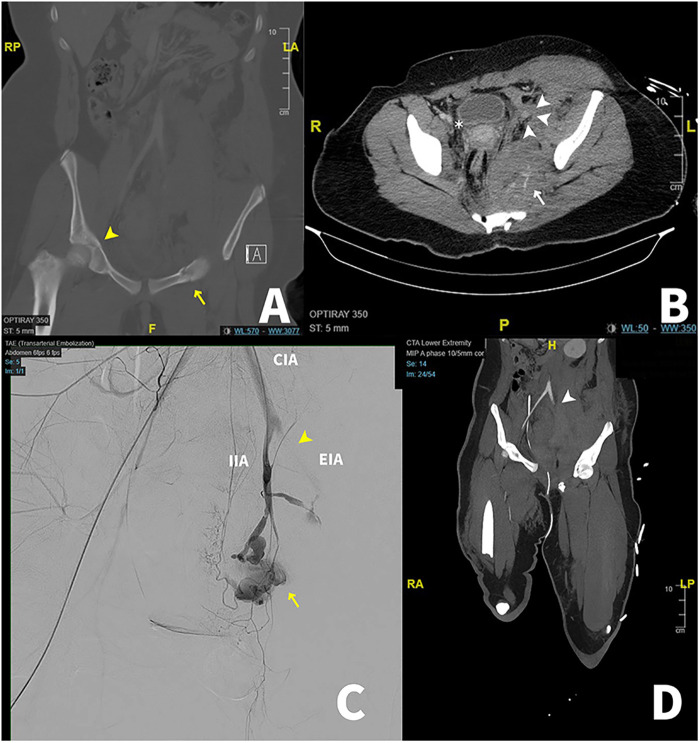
Initial contrast-enhanced computed tomography (CT) scan (A,B), transarterial embolization and subsequent emergent computed tomography angiography (C,D). (A) Bone window of the scan. The yellow arrowhead indicates a left pubic ramus fracture. The yellow arrow indicates a right acetabular fracture. (B) The white arrow indicates contrast extravasation from the left pelvic wall. The white asterisk marks the normal right external iliac artery and vein. The white arrowheads indicate a compromised left external iliac artery. (C) Angiography showing labeled vessels: common iliac artery (CIA), IIA and EIA. The yellow arrow indicates contrast extravasation. The yellow arrowhead indicates the initially neglected obstructed left external iliac artery. (D) The white arrowhead indicates a completely obstructed left external iliac artery.
